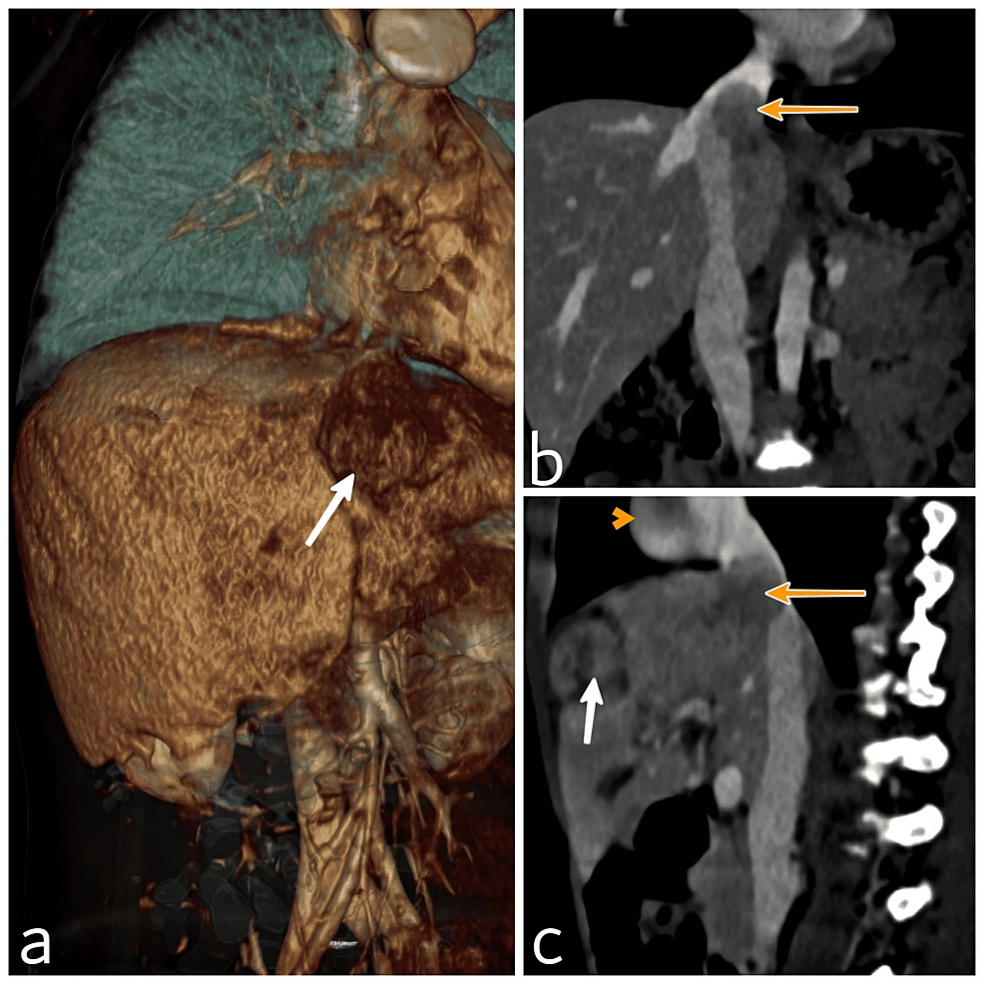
CECT images. (a) 3D volume reconstruction showing the tumor in the left lobe of liver (white arrow). (b) Coronal reconstructed section showing tumor thrombus in the IVC (yellow arrow), (c) Sagittal reconstructed section showing the tumor (white arrow), IVC thrombus (yellow arrow), and thrombus in the right atrium (yellow arrowhead). CECT - Contrast-enhanced computed tomography
